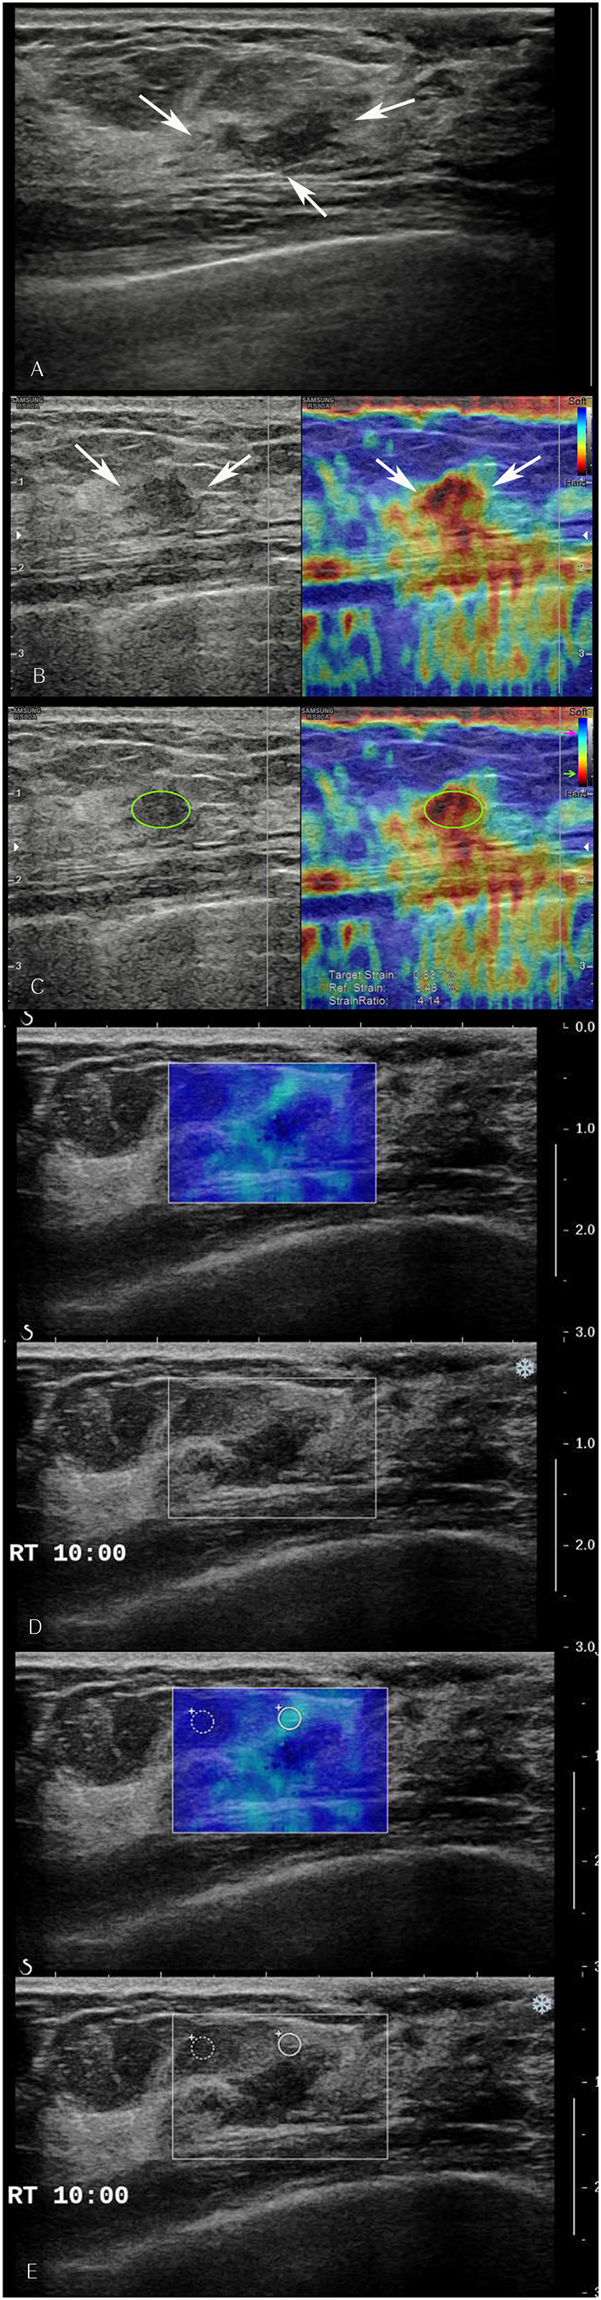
An abnormality detected via screening mammography in a 50-year-old woman. (A) Grayscale ultrasound shows an indistinct irregular isoechoic mass (arrows) in the 10 o’clock area of the right breast which was categorized as a category 4a tumour with low suspicion of malignancy. (B) By strain elastography, the mass was graded with a visual score of 5 according to the Tsukuba system (arrows). (C) Strain ratio was 4.1. (D) By shear wave elastography, the mass was graded with a colour score of 3. (E) Elasticity ratio was 5.41. Ultrasound core biopsy confirmed the tumour to be a ductal carcinoma *in situ*.
